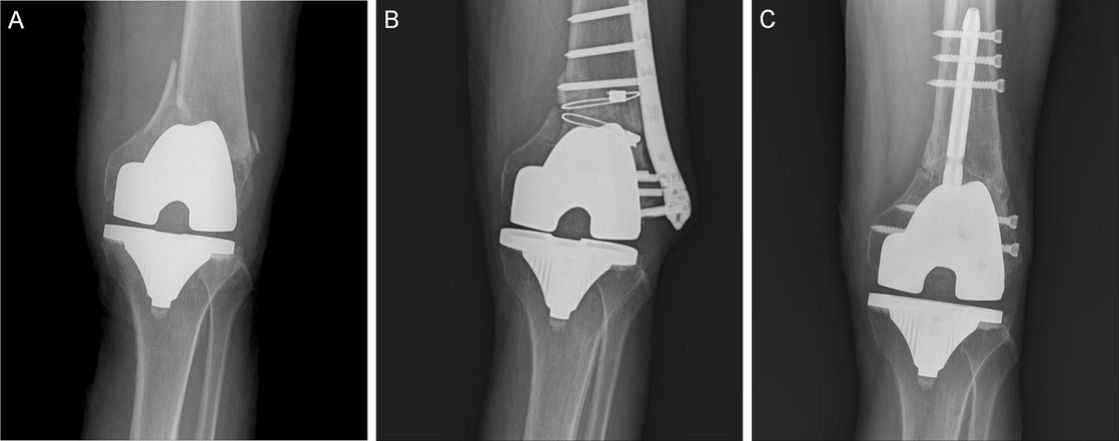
a Case 1. Preoperative X-rays of a periprosthetic fracture of the *left* knee in a 75-year-old man. b Radiograph of metal failure with the FP system taken 6 months later. c Follow-up radiograph taken 2 years later after fracture fixation with the RISN showing callus formation
